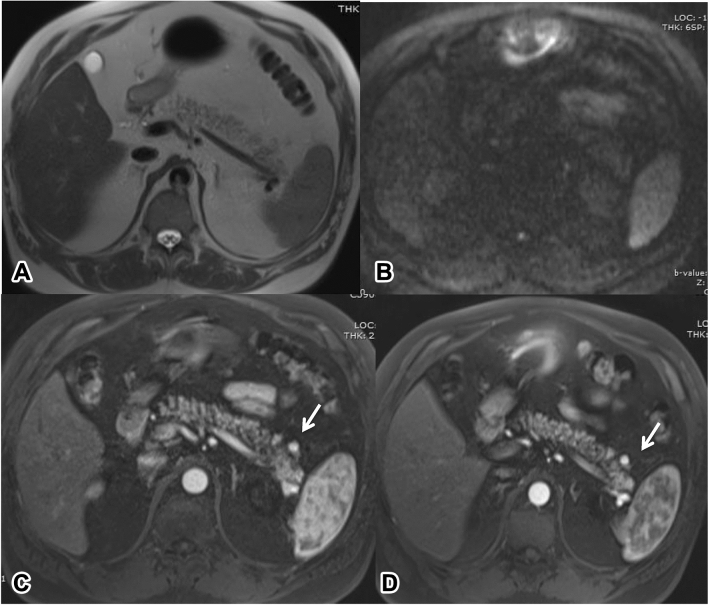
A–D: small PNET (< 10 mm). On T2 HASTE (A), and DWI (b 800: B), no lesion with pathologic features is appreciable. In the complete workout, a small hypervascular lesion is appreciable during arterial phase (C) after Gadobenate Dimeglumine (Multihance, Milano, Italy). The lesion is unchanged in comparison to a previous study, 1 year before (D)
